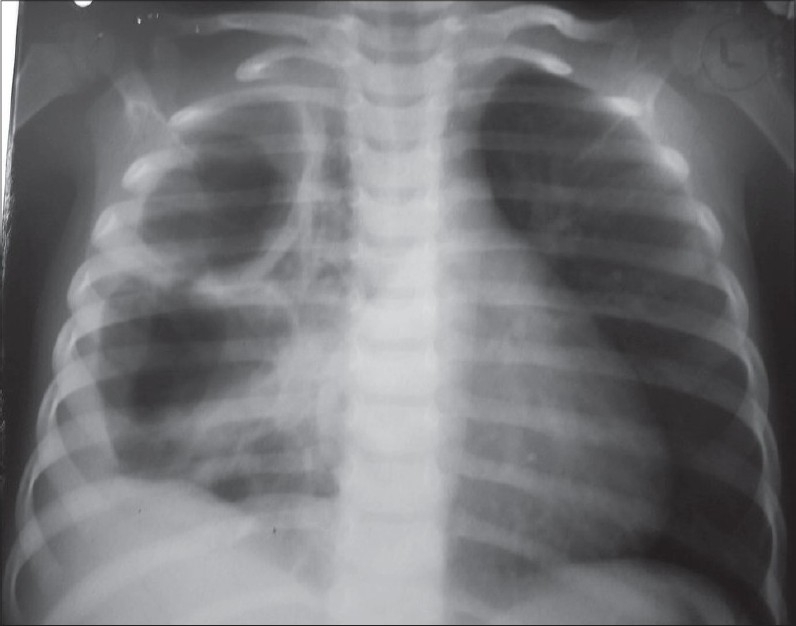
Chest X-ray showing multilple loculations in right hemithoraxCavitary lung necrosis [Figure 7]Persistent broncho-pleural fistula with collapse of lung.
